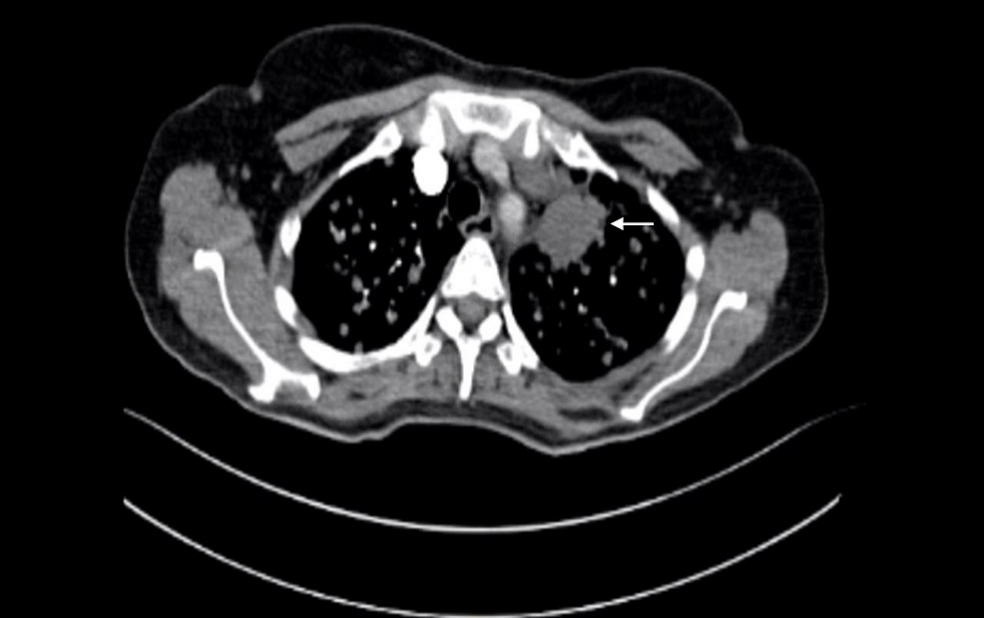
CTPA revealed many diffuse bilateral pulmonary nodules measuring up to 1.5 cm and an ill-defined mass with speculated margins noted in the left upper lobe (white arrow). CTPA: computed tomography pulmonary angiogram
